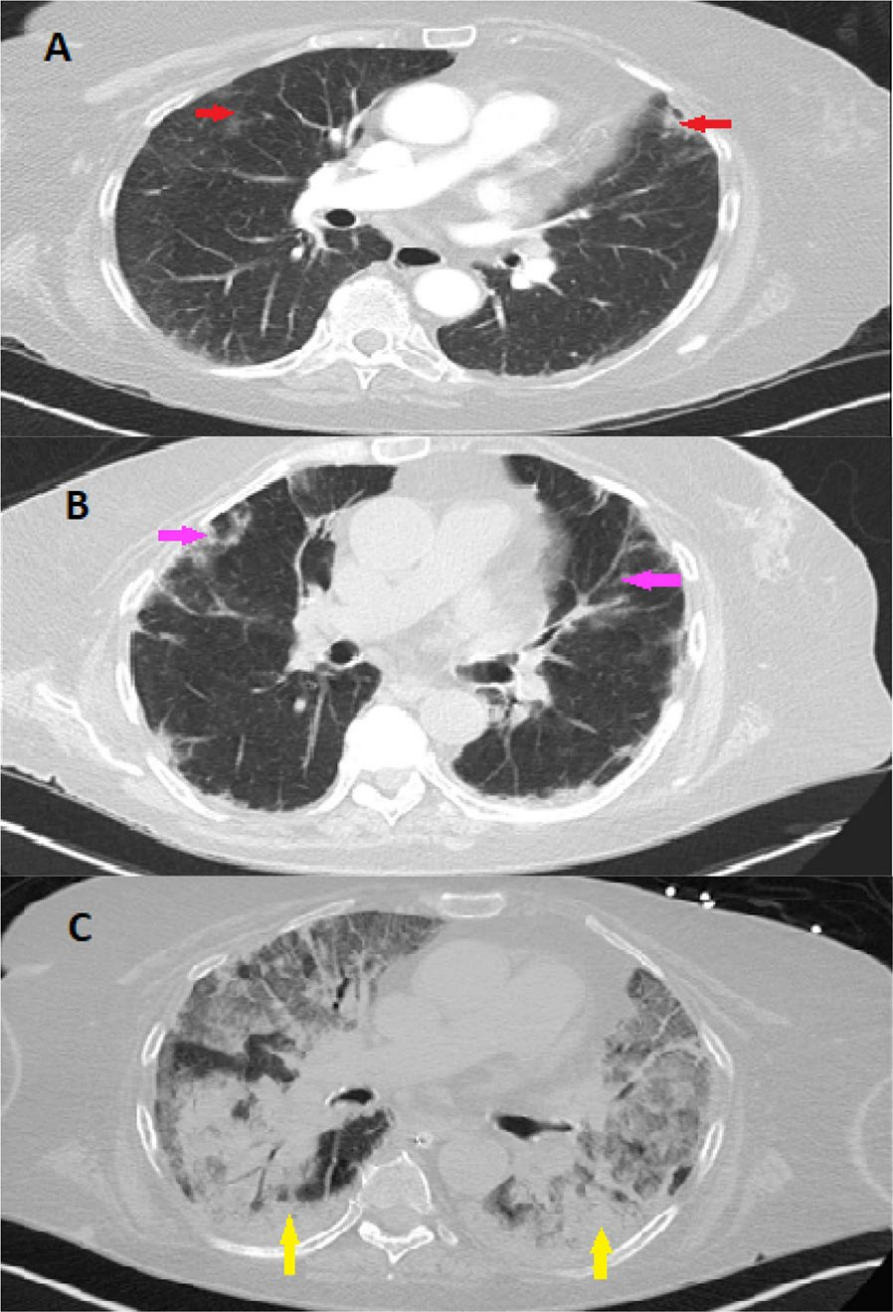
Computed Tomography of the Chest. CT scan of the chest with contrast obtained during the first admission demonstrates mild bilateral patchy infiltrates showing by red arrows (a). CT of chest obtained during second admission demonstrates bilateral patchy ground glass densities as showing by pink arrows (b). CT of chest during the patients second hospital admission demonstrates worsening consolidative processes within the bilateral lower lobes, more prominently in a peripheral distribution (yellow arrow, c)
